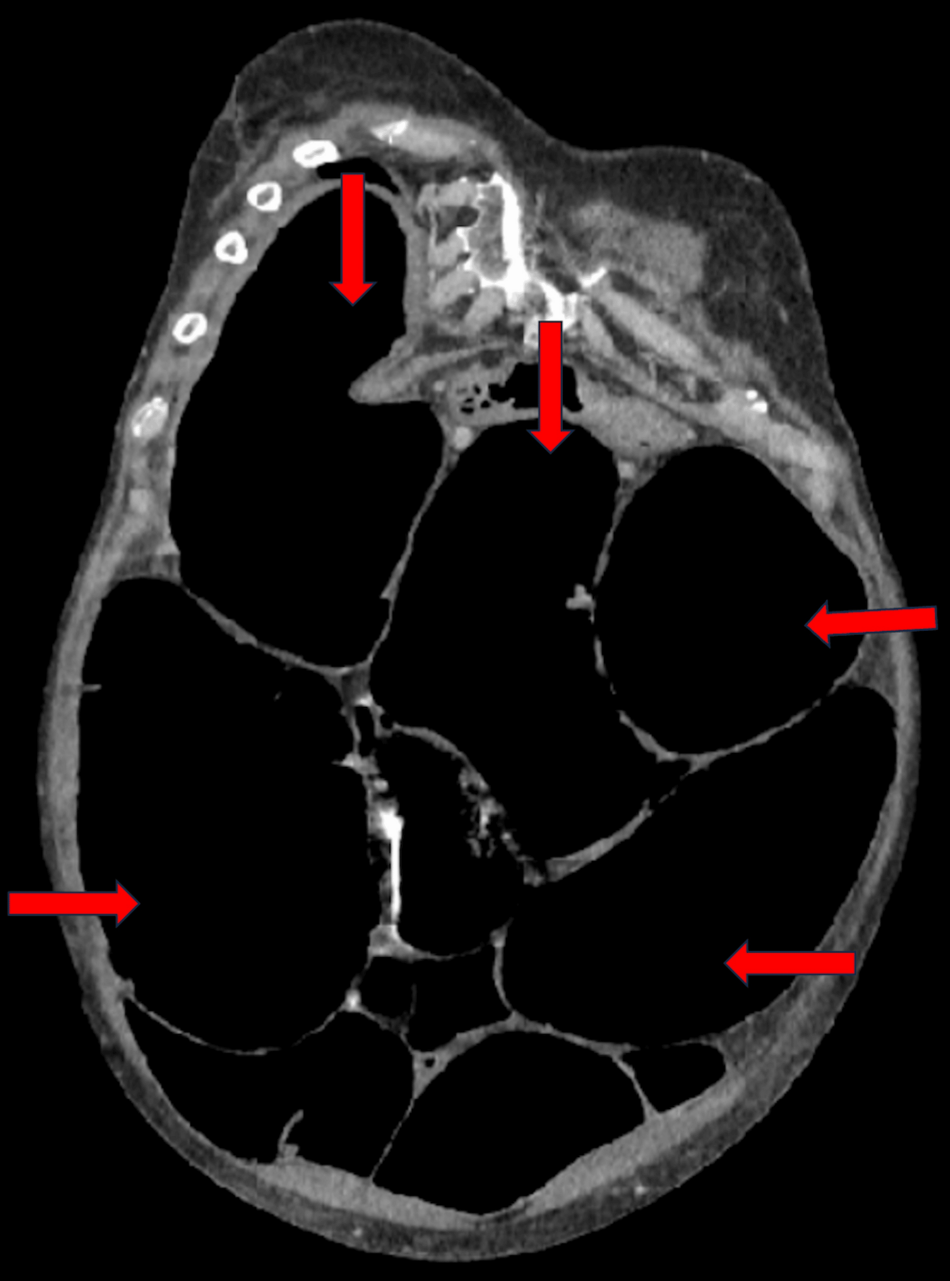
Coronal CT of the abdomen demonstrating multiple loops of large bowel that are significantly dilated (red arrows).
